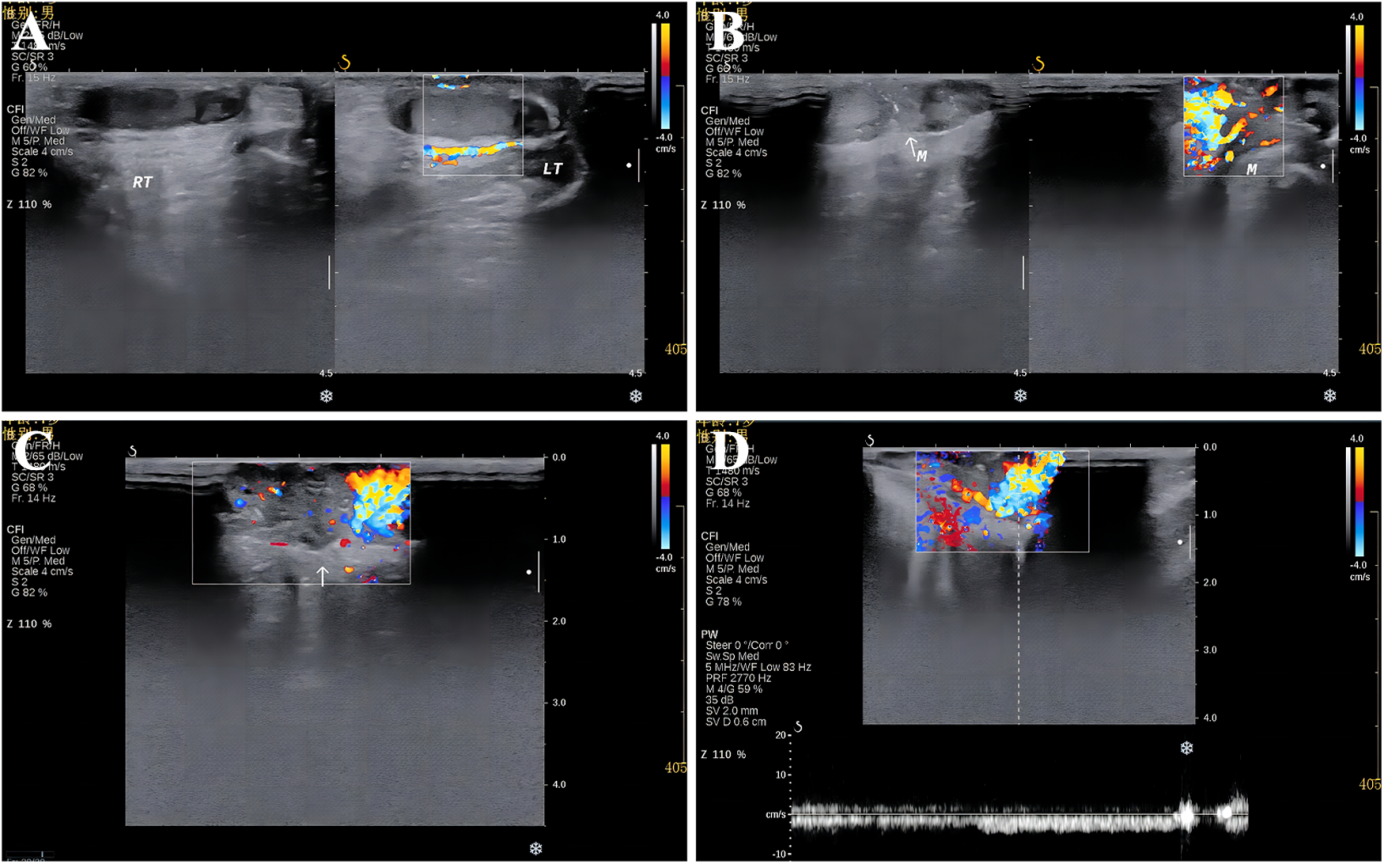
Preoperative colour Doppler ultrasonography; (A) bilateral testicular colour Doppler ultrasonography; (B,C) giant scrotal mass detected by CDFI; (D) findings on PD examination of the giant scrotal mass.
